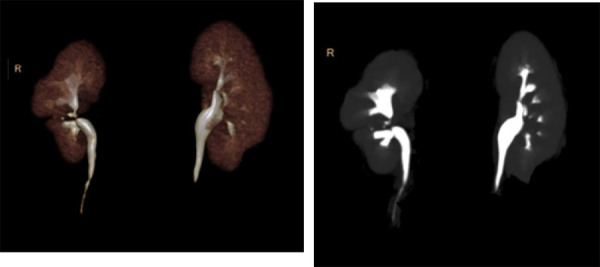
3D reconstruction (A) and maximum intensity projection (B) of CT urography image in excretory phase showed a separately draining calyceal system of caudal right supernumerary kidney uniting with calyceal system of cranial right kidney at the extrarenal pelvis and having a single common right ureter.
